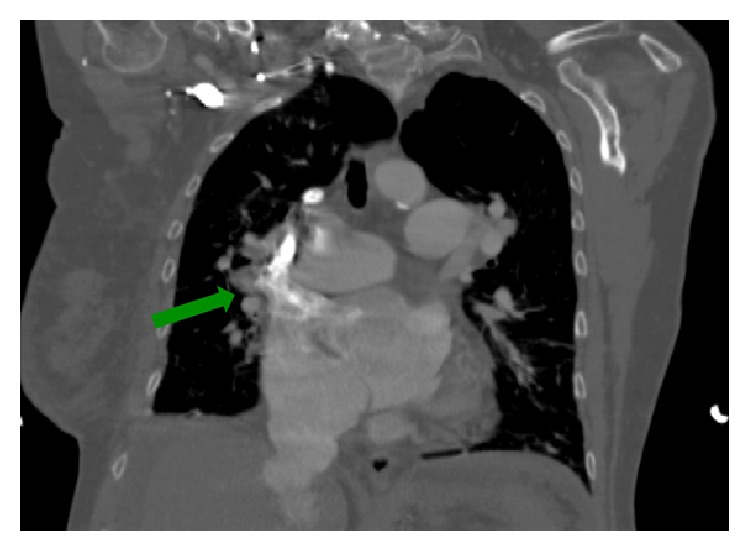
CT-scan showing anomalous right upper pulmonary venous return to superior vena cava (green arrow).
